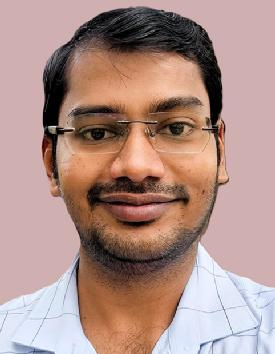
Dr Abhishek Kumar, with a Doctor of Medicine (DM) degree in Cardiology, is working as an Assistant Professor at the Department of Cardiology, Government Superspeciality Hospital CIMS, Koni, Bilaspur, India, which is a government tertiary healthcare and research institute. His research focuses on critical areas such as acute heart failure, high-sensitivity cardiac troponins, and strain echocardiography, contributing valuable insights to the field with multiple published papers. His clinical expertise includes proficiency in primary angioplasty, pacemaker implantation, and structural cardiac interventions, making him a well-rounded practitioner.
